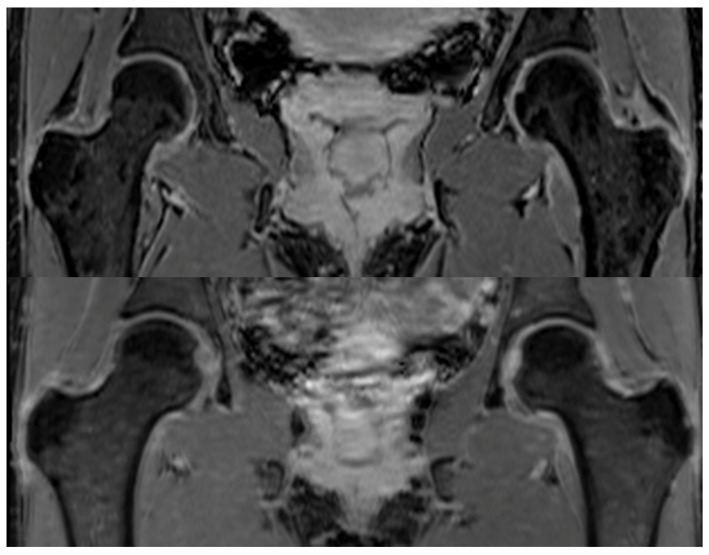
Two examples of DIXON MRI sequences highlighting the left and right femurs and the acetabulum of a patient (top) and a healthy subject (bottom). Both images were acquired using an axial dual-echo T1-weighted 3D FLASH sequence with Dixon fat–water separation, enabling detailed visualization of hip joint structures. Although the image contrast appears visually similar between the two cases, the symptomatic patient exhibits a subtle but clinically relevant narrowing of the space between the femoral head and acetabulum. These morphological changes are difficult to appreciate in isolated 2D views, and this challenge underlies the motivation for the current study, which leverages radiomic features to detect and quantify such structural differences objectively.
